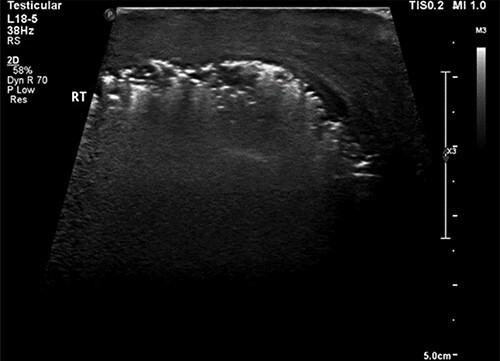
Ultrasound image of the right hemiscrotum showing gas bubbles causing acoustic shadowing and ringdown reverberation artifacts obscuring the deep tissue namely testicular parenchyma.
